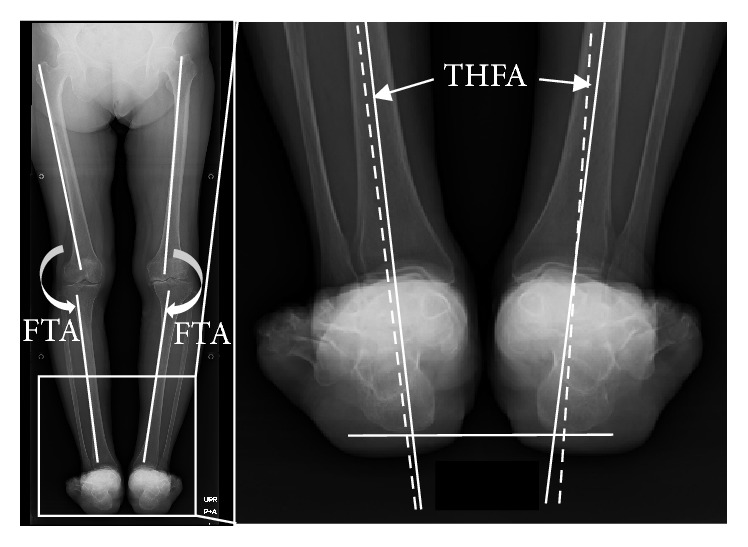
The hip-to-calcaneus (H-to-C) radiograph is shown in the left and the FTA was determined as shown. In the right radiograph, there is an enlargement of the left radiograph, the THFA, which is the internal angle of the tibial axis (solid white line). The dotted white line between the center of the talar dome and the lowest point of the calcaneus was measured.
